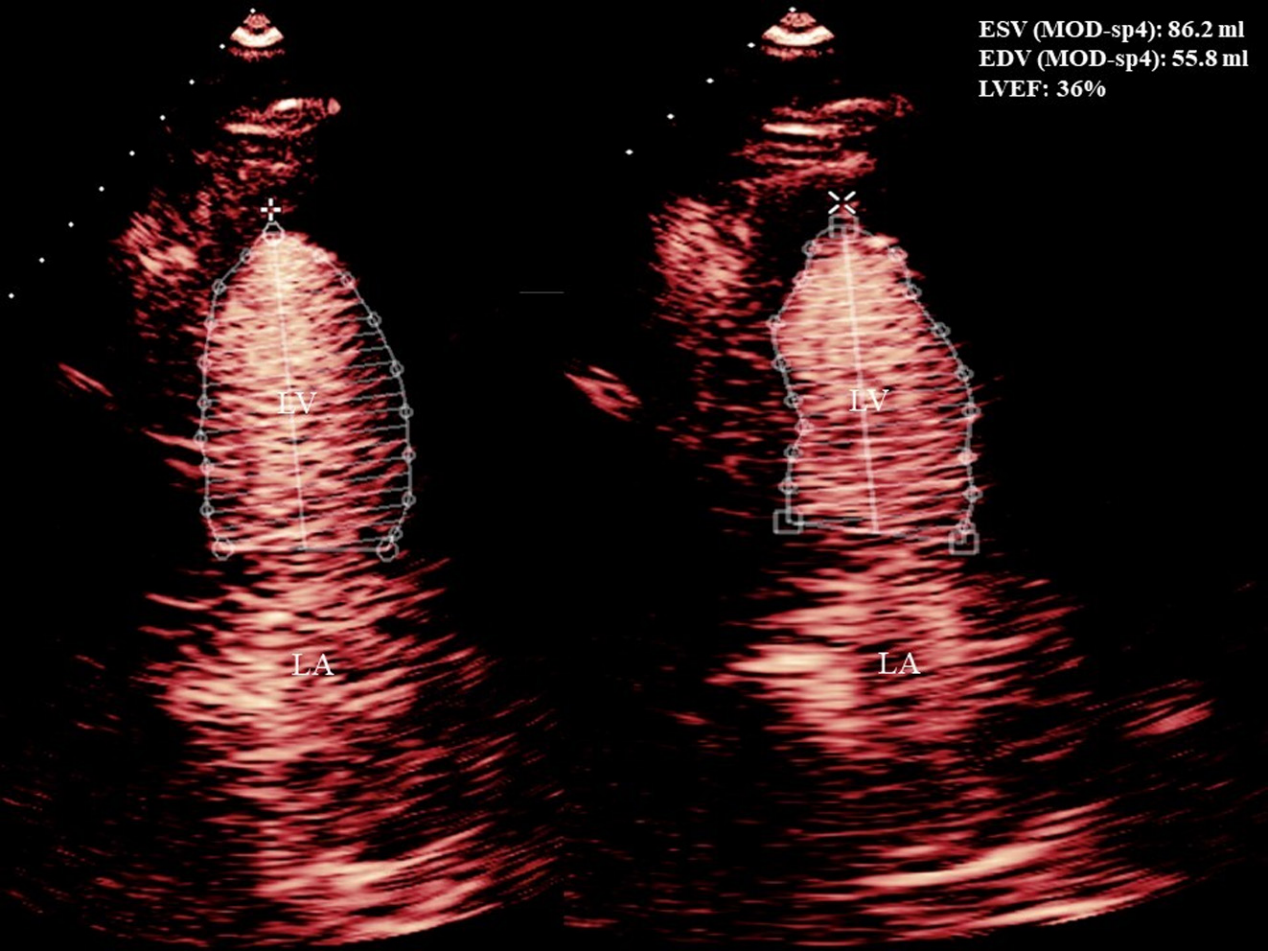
Contrast transthoracic echocardiography showing reduced left ventricular systolic function with an estimated left ventricular ejection fraction of 35%.
